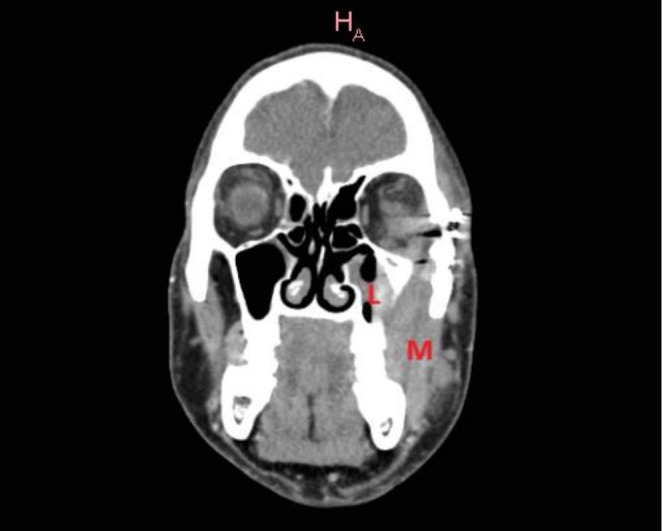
Computed tomography (CT) scan shows (L) a left maxillary soft tissue mass and (M) enlargement of the masticator space.
